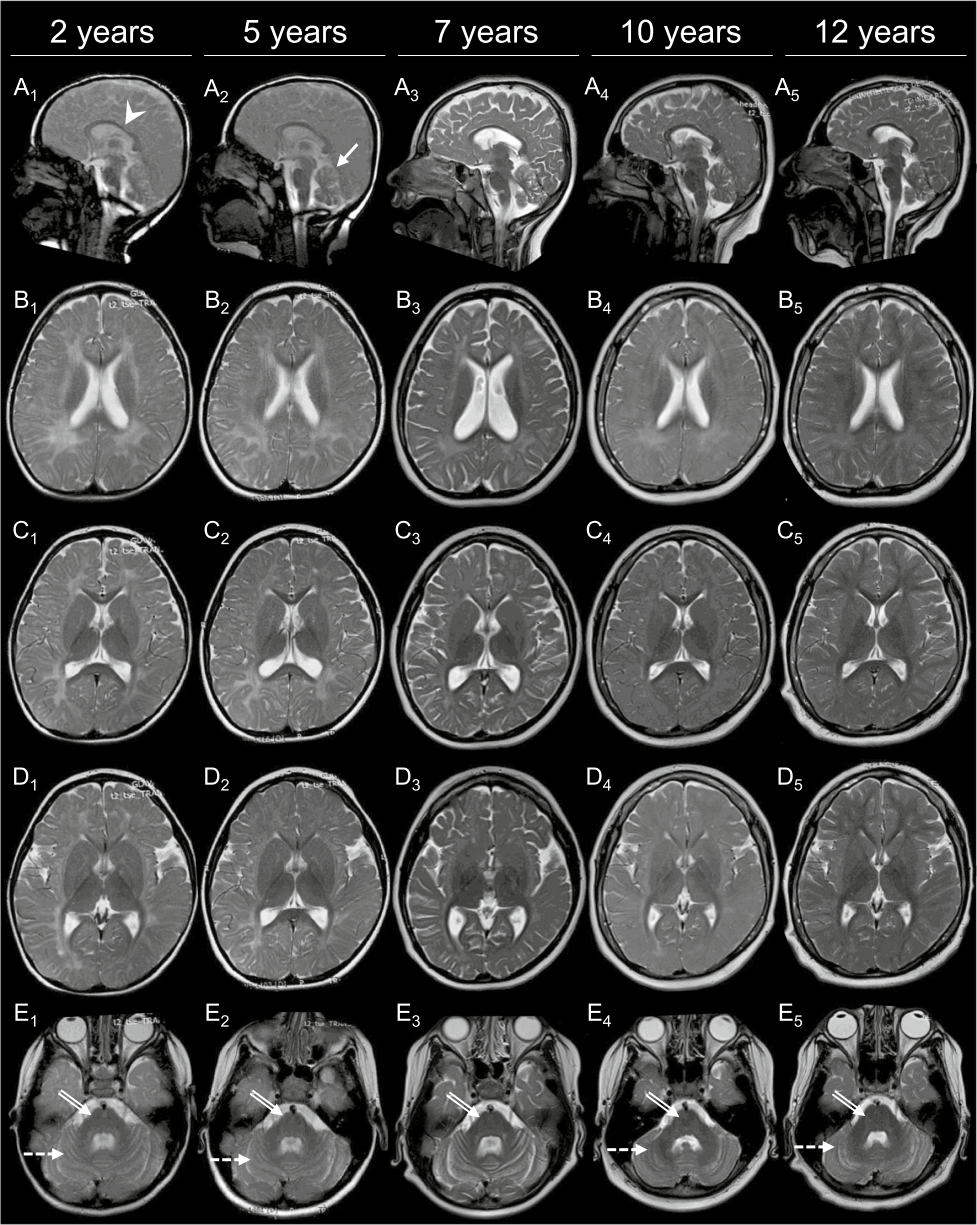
Brain MRI of Patient 1 from age 2 to 12 years. T2-weighted images are shown at 2 years (column 1: A_1_–E_1_), 5 years (column 2: A_2_–E_2_), 7 years (column 3: A_3_–E_3_), 10 years (column 4: A_4_–E_4_) and 12 years (column 5: A_5_–E_5_). Sagittal (panel A) images show mild to moderate thinning of the corpus callosum (white arrowhead), as well as mild cerebellar atrophy (white arrow). In panels B_1–3_, C_1–3_, and D_1–3_, severe lack of myelin deposition, together with progressive cerebral atrophy are appreciated. In panels B_4–5_, C_4–5_, and D_4–5_, improvement in myelination is seen, but incomplete myelination is still present at age 12 years. Of note, brain volume has improved at ages 10 and 12 years (B_4–5_, C_4–5_, and D_4–5_). In panel E, insufficient myelin deposition is seen in E_1-2_ in both the pons (white double-lined arrow) and cerebellum (white dashed arrow), with improvement in the pons at age 7 years (E_3–5_, white double-lined arrow) and significant improvement in the cerebellum at ages 10 and 12 years (E_4–5_, white double-lined arrows). Progressive cerebellar atrophy is also seen between ages 2 and 7 years (E_1–3_), with improvement in subsequent MRIs done at ages 10 and 12 years (E_4–5_)
